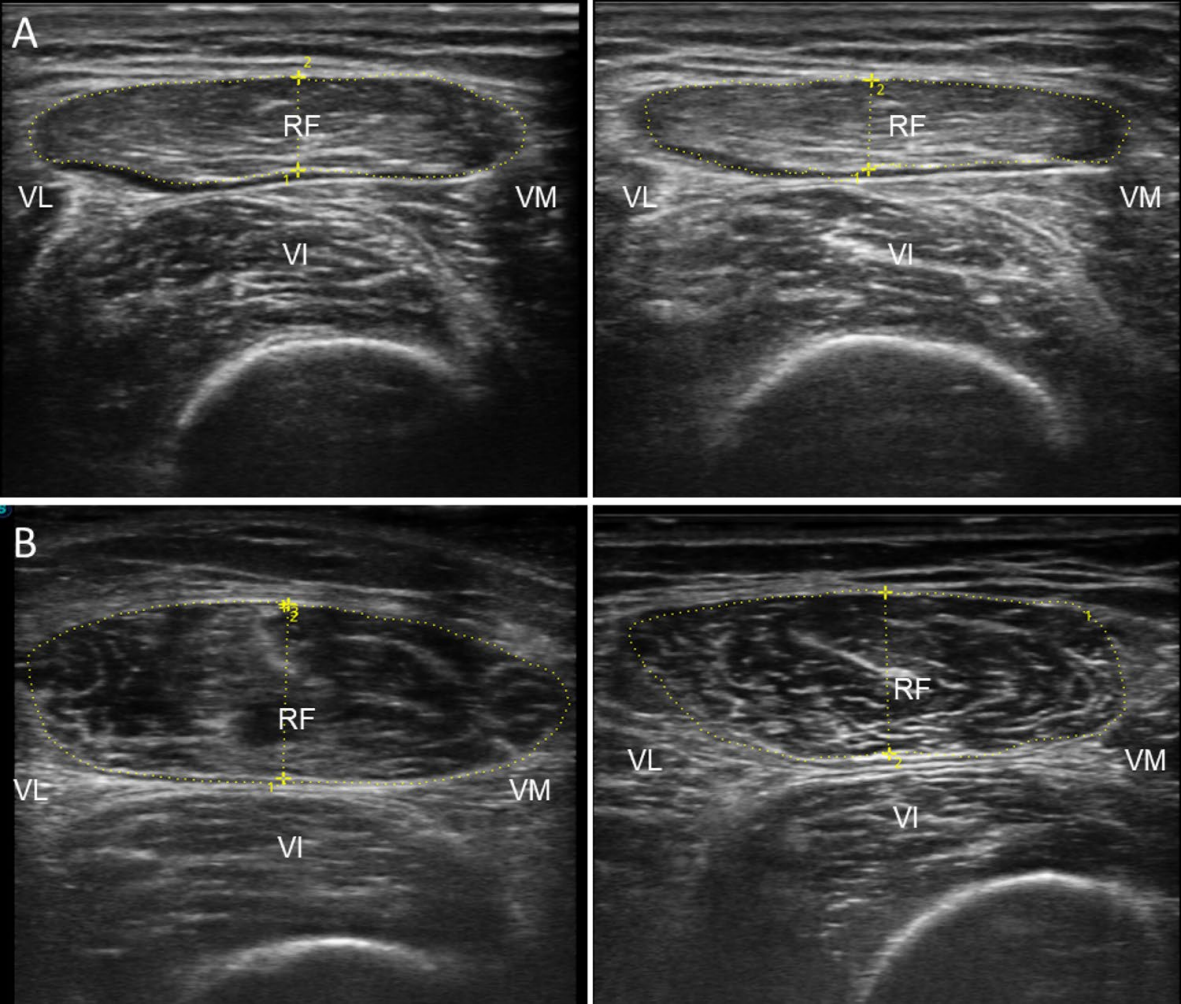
Muscle Ultrasound of Patients at Baseline and after 72 h. A and B Indicate ultrasounds of different patients, left panel ultrasounds at the baseline and right panel after 72 h—*RF* Rectus Femoris, *VI* Vastus Intermedius, *VL* Vastus Lateralis, *VM* Vastus Medialis
